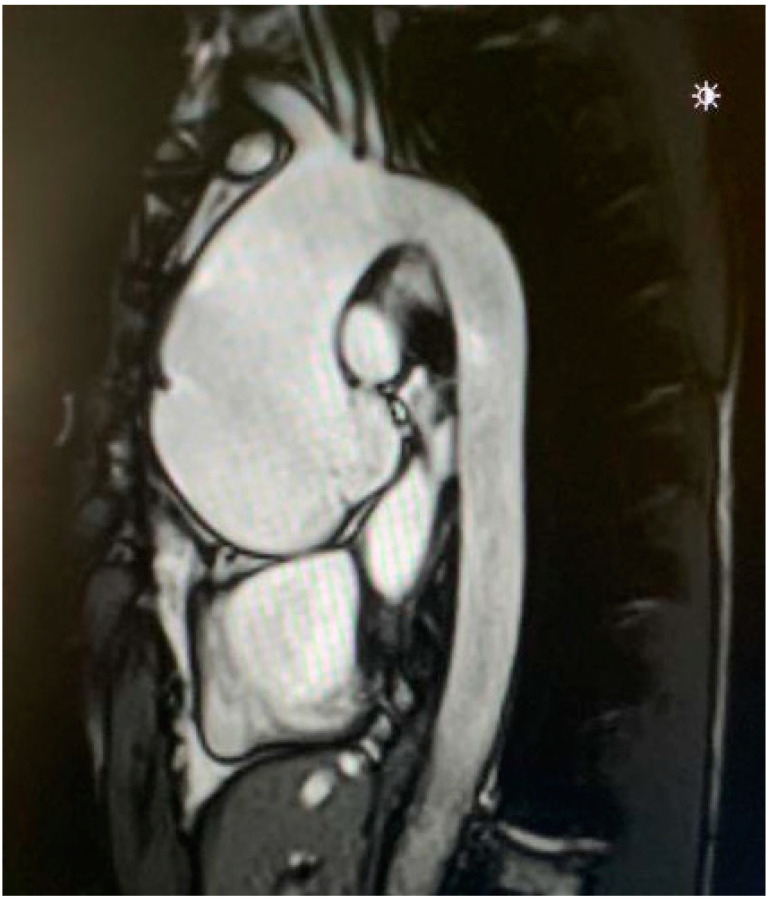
Cardiac magnetic resonance imaging showing a severe aortic root dilation in a patient with Marfan syndrome.
